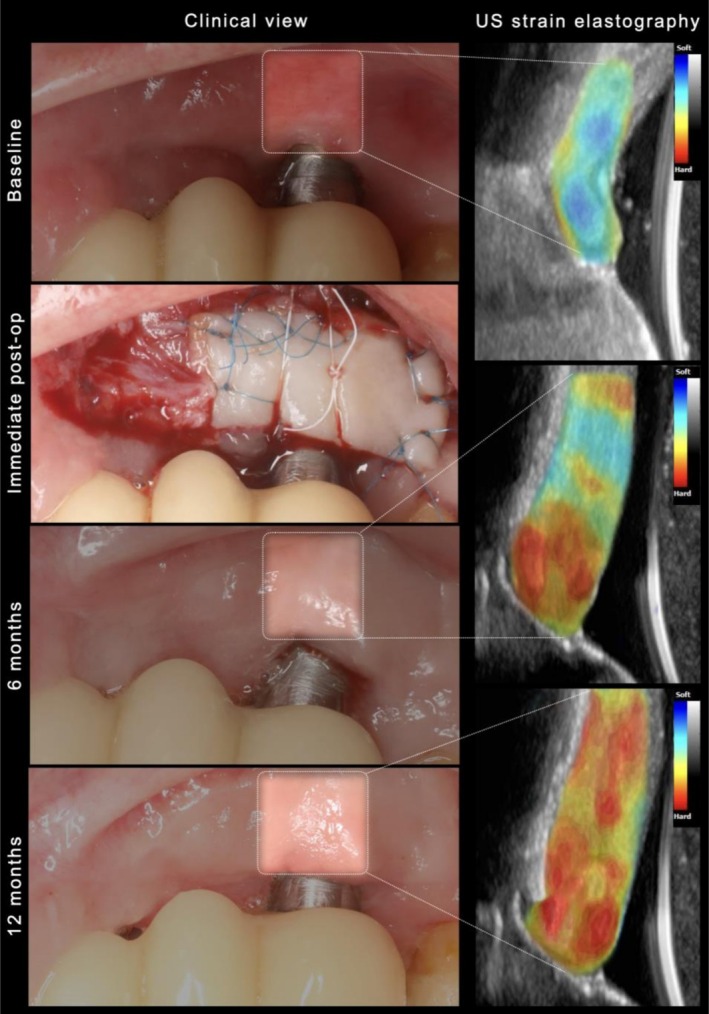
Changes in ultrasonographic (US) tissue elasticity following APF + FGG on an isolated posterior maxillary implant. Orange and red colors in the color‐coded maps (elastograms) indicate that the region of interest is hard/stiff, while colors towards light blue and blue denote that the region of interest is soft/elastic. It is possible to appreciate the increase in stiffness of the grafted area, especially in the coronal portion of the soft tissue, at 6 months and, even more, at 12 months.
